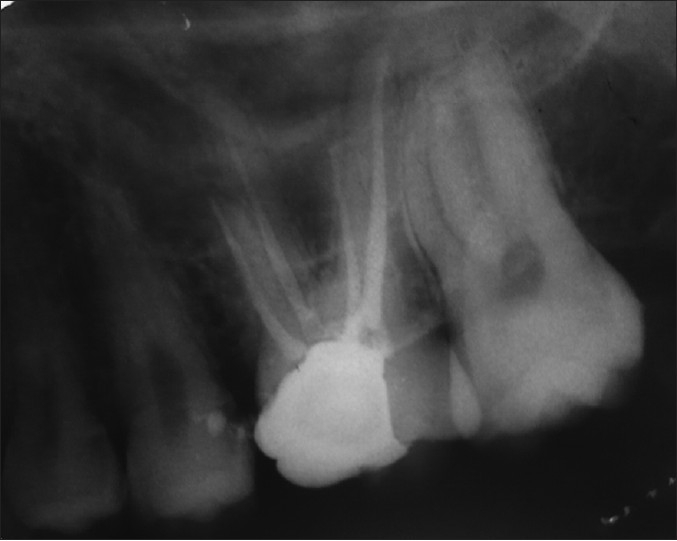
Post obturation radiograph of 16
